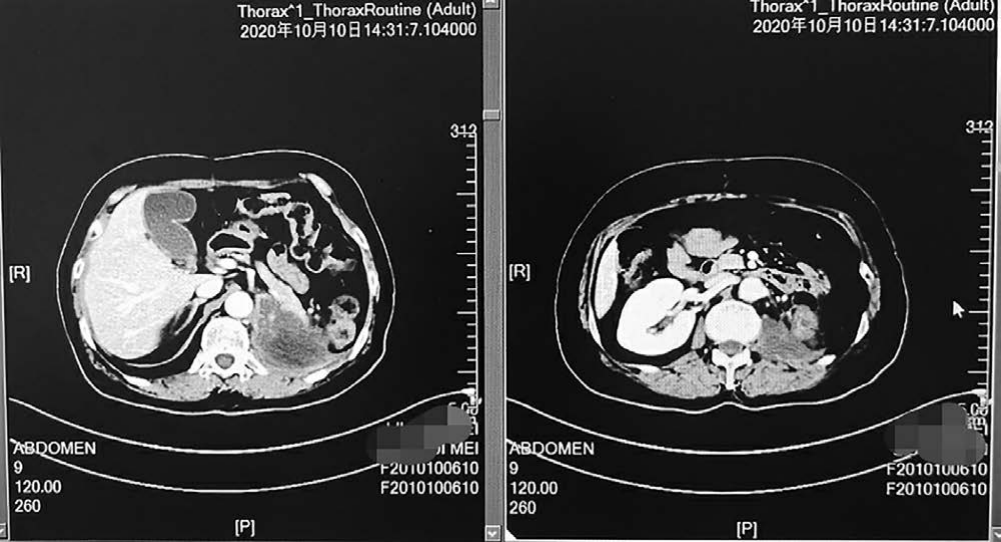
Computed tomography shows the lesions in the abdominal cavity before the regimen of ‘tislelizumab plus apatinib’ was given.
